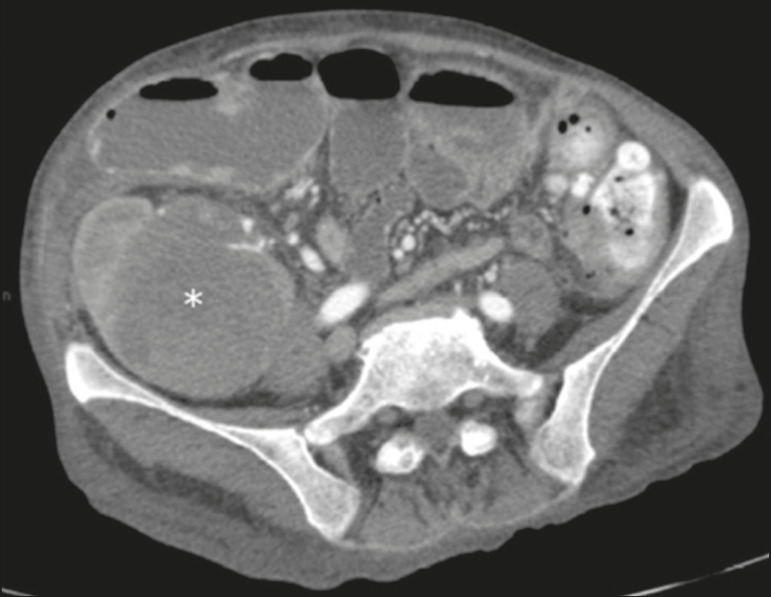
Contrast-enhanced axial CT of the abdomen, in the arterial phase, showing, at the level of the renal graft, a solid, slightly heterogeneous mass that is hypodense in relation to the renal parenchyma (asterisk), which was indicative of lymphoproliferative disease, after transplantation.
